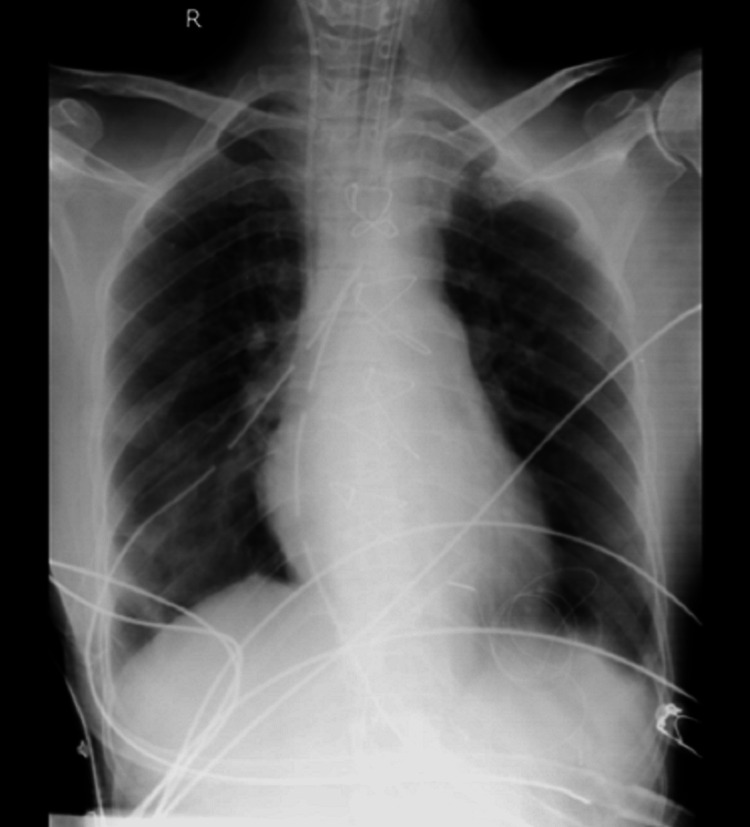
Postoperative chest x-ray PA view PA: posterior-anterior
